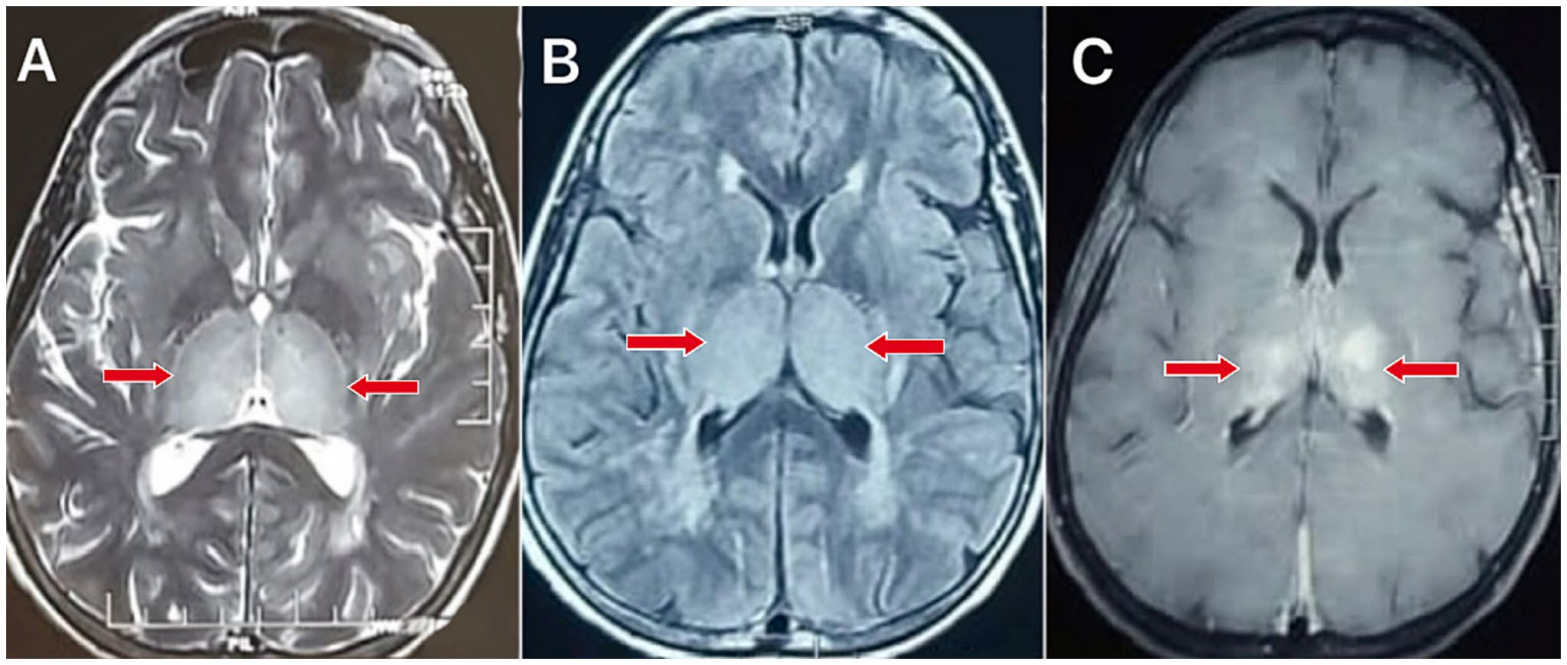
Axial MRI images of the brain demonstrate bilateral symmetrical lesions (indicated by red arrows) in the thalamus across different sequences: (A) T2-weighted image shows hyperintense lesions in the thalamus. (B) Fluid-Attenuated Inversion Recovery (FLAIR) sequence reveals prominent hyperintense lesions in the thalamus. (C) Diffusion-weighted imaging (DWI) sequence shows restricted diffusion in the thalamic lesions.
